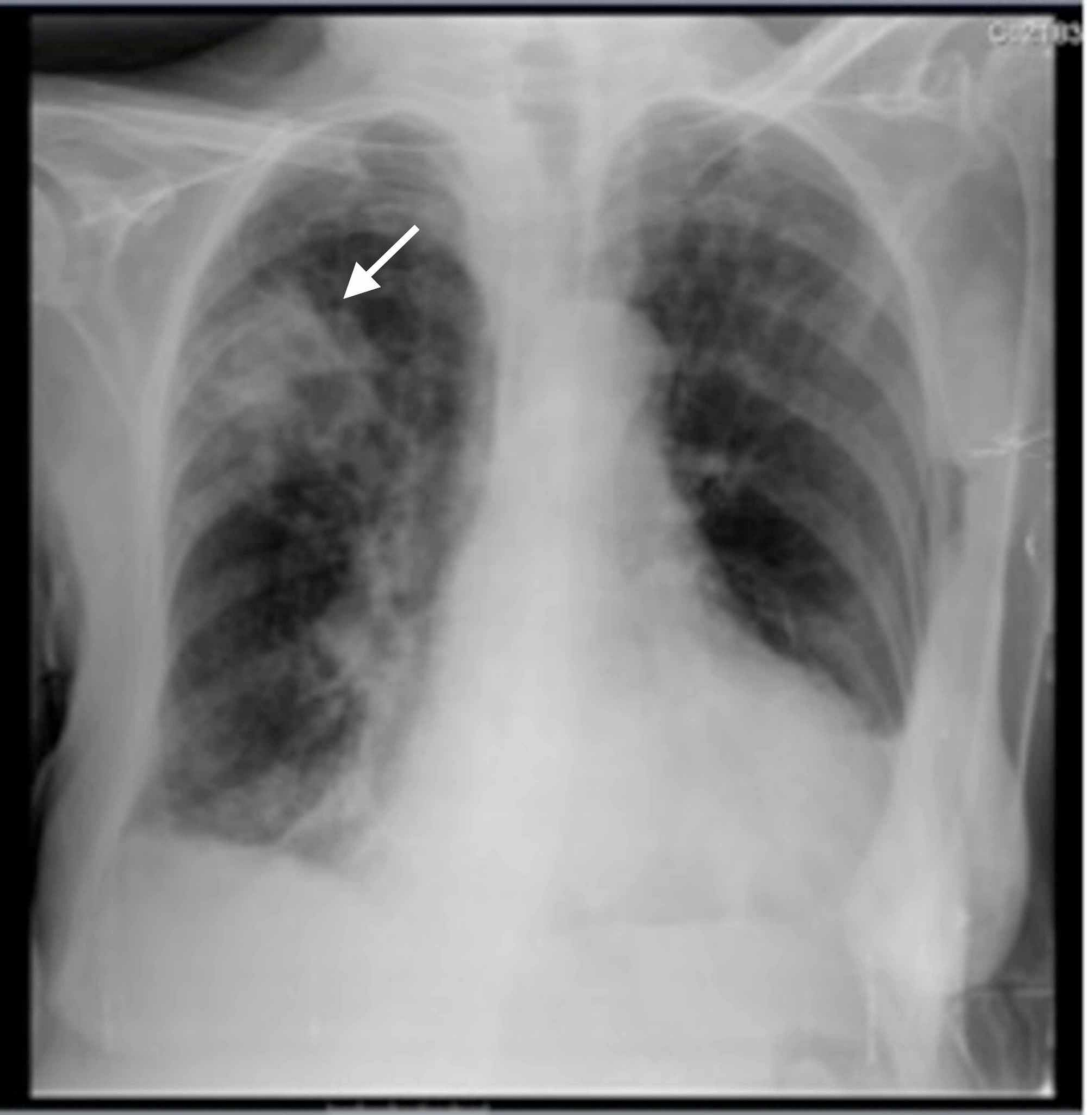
Chest X-ray Condensation image in the right upper lobe (arrow).
